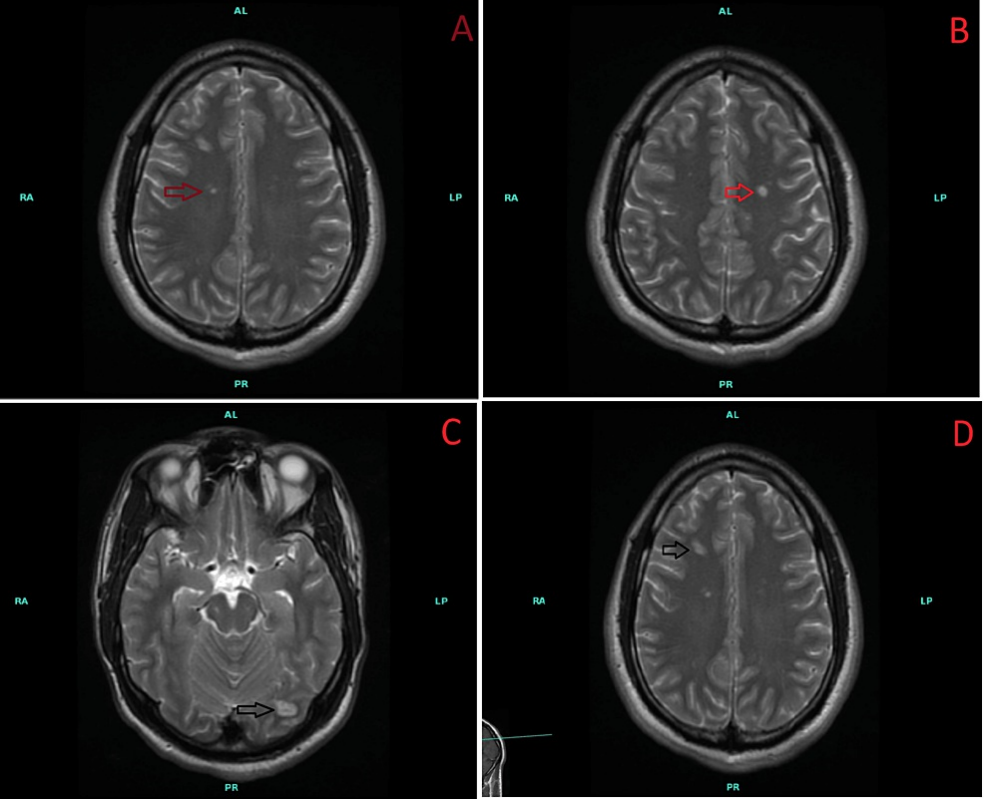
MRI brain findings showing bilateral cerebral multifocal acute non-hemorrhagic infarcts (red arrows in A and B) The largest on the left measures 4.0 x 1.8 cm on the occipital lobe subcortical white matter (black arrow in C). The largest on the right measures 1.0 x 0.5 cm on the frontal lobe subcortical white matter (black arrow in D).
